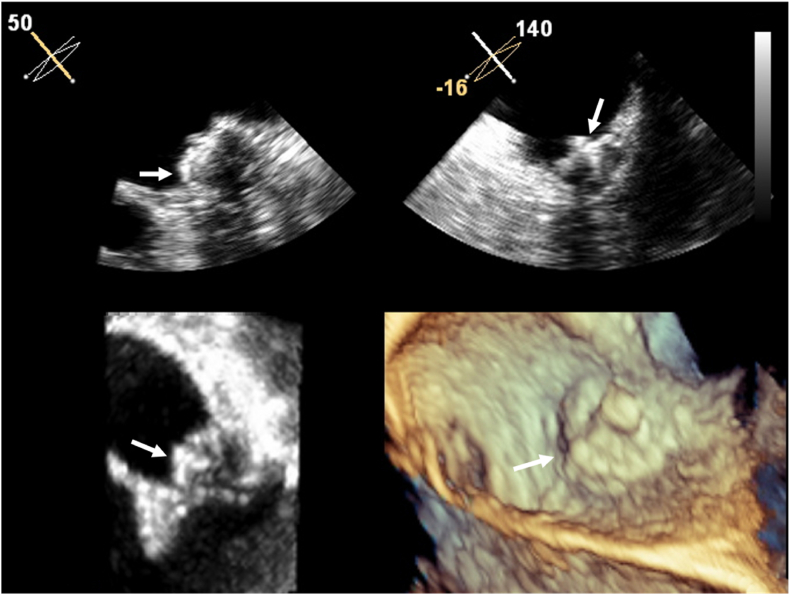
Transesophageal echocardiography seven months post implantation showing thrombus resolution.
